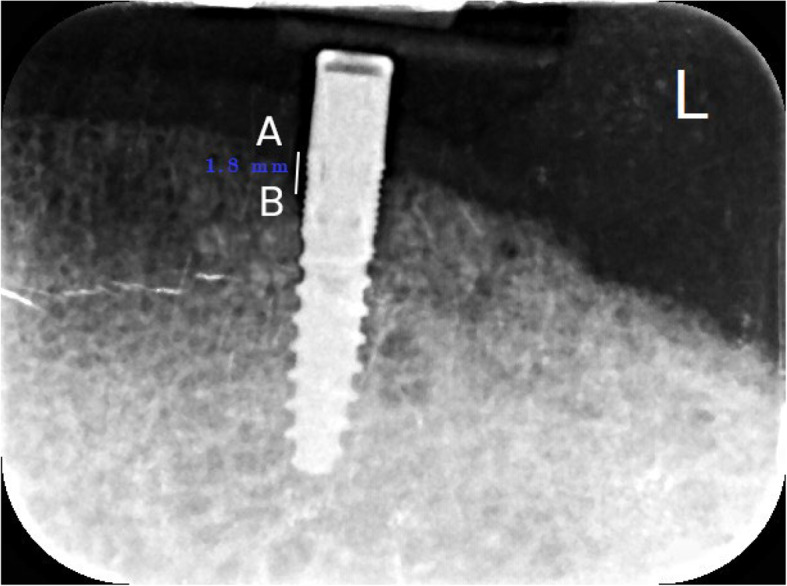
Periapical radiograph of implants. A: represents the implant shoulder, B: represents the initial bone-to-implant contact
